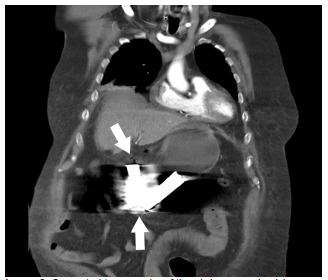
Computed tomography of the abdomen and pelvis demonstrating foreign bodies that appear to be in the stomach with extensive artifact due to metallic foreign bodies. Bottom arrow points to two foreign bodies, which were reported to be two AA batteries per the patient. Top arrow points to free air within the peritoneum suggesting hollow viscus perforation.
